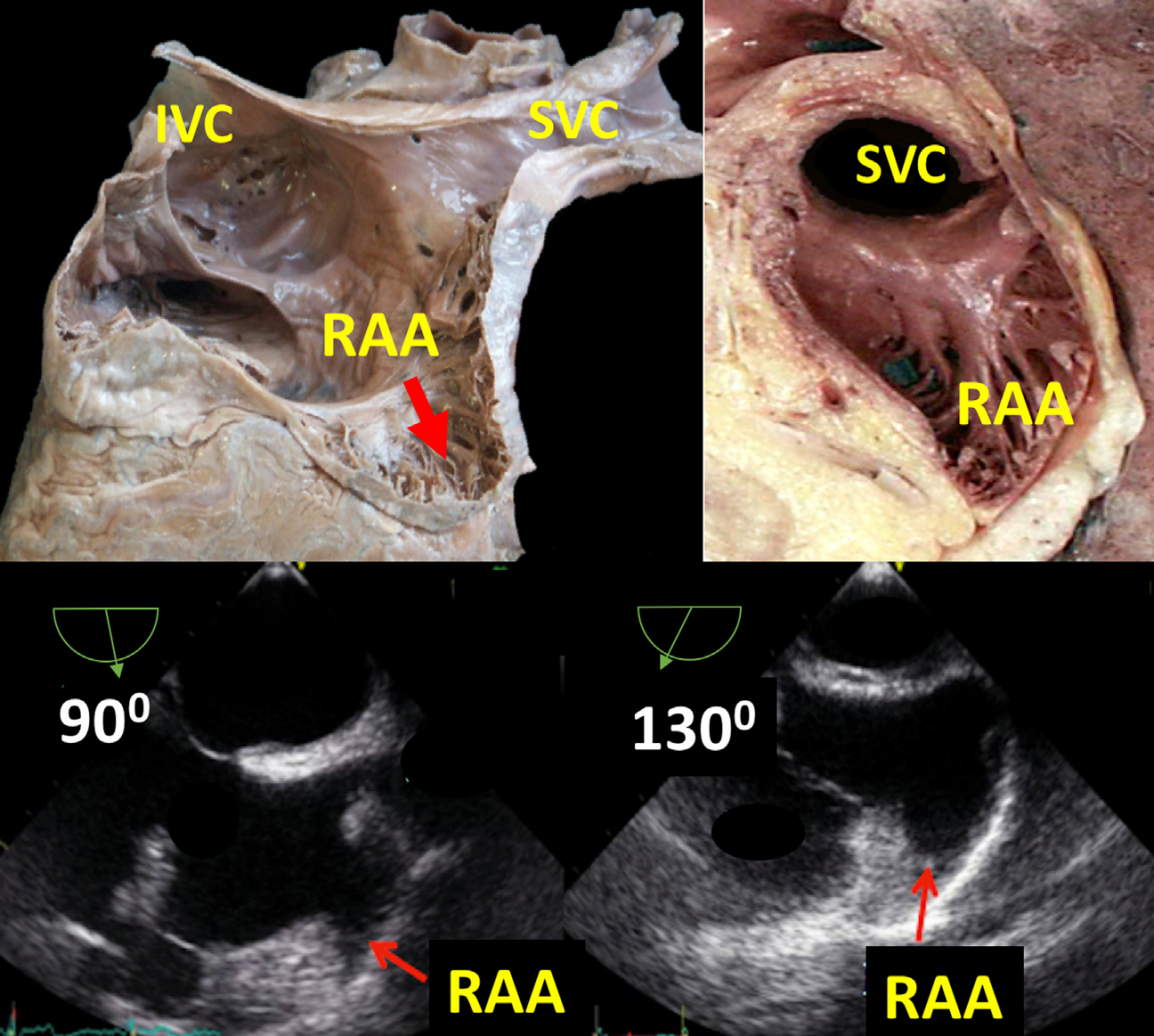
Anatomic and Transesophageal Echocardiographic View of Midesophageal Bicaval View at 90° and 130° of the Right Atrial Appendage IVC = inferior vena cava; RAA = right atrial appendage; SVC = superior vena cava.
